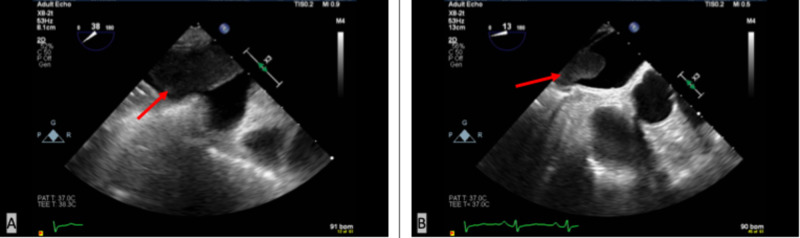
Trans-esophageal echocardiogram. A: a tumor thrombus extending through the right inferior pulmonary vein (red arrow); B: left atrial tumor thrombus (red arrow).
